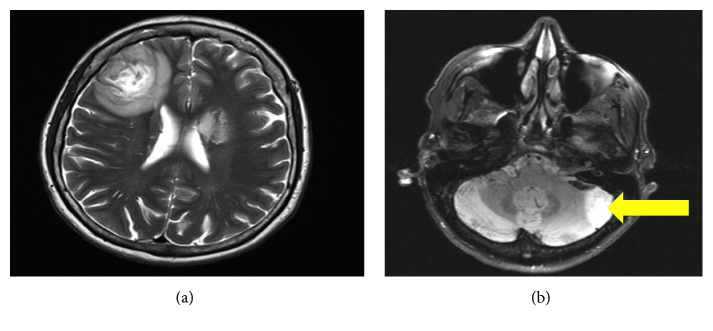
(a) CT head with contrast showing a 3 cm region of heterogeneous low density in the right frontal lobe. A second, 1.5 to 2 cm region of low attenuation in the anterior aspect of the left basal ganglia consistent with an intraaxial mass lesion compressing the left lateral ventricle. (b) MRI brain with contrast showing a central cavitary, contrast-enhancing lesion involving the lateral left cerebellar hemisphere (see arrow).
